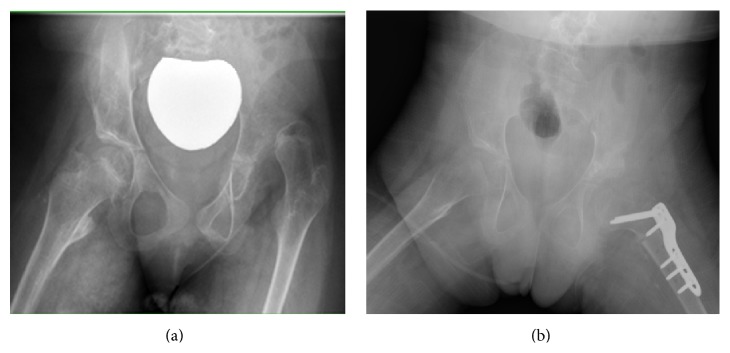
Preoperative and postoperative X-rays of a dislocated hip in a child with cerebral palsy. The left X-ray showed a dislocated left hip in a child with CP. The acetabulum has become shallow and is not covering the femoral head any more. There is a valgus deformity of the proximal femur. The postoperative pictures showed that the hip is reduced, the proximal femoral deformity is corrected (varus derotation corrective osteotomy), and the acetabulum is corrected by pelvic Dega osteotomy.
